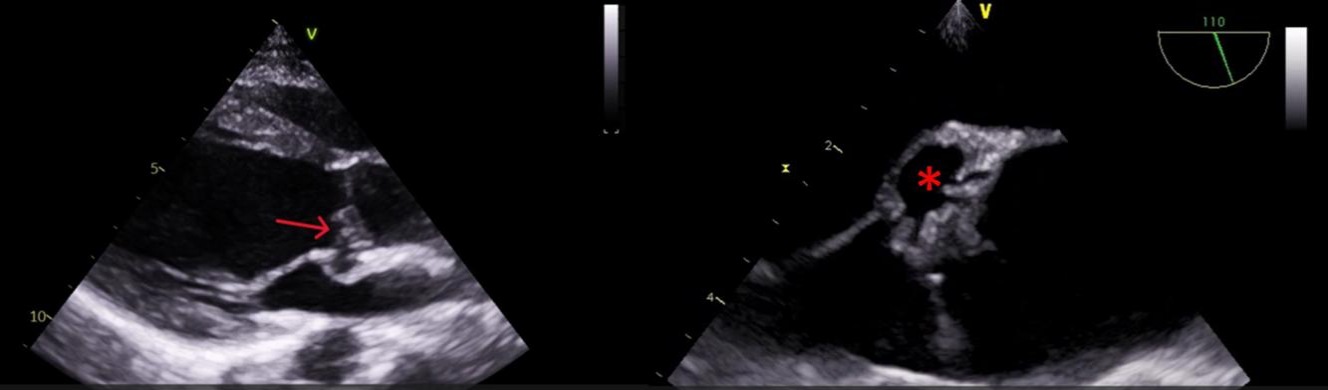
Parasternal long-axis transthoracic view of aortic valve vegetations marked by arrow (left). Mid-oesophageal long-axis view focussing on aortic valve demonstrating abscess cavity marked by asterisk (right).
